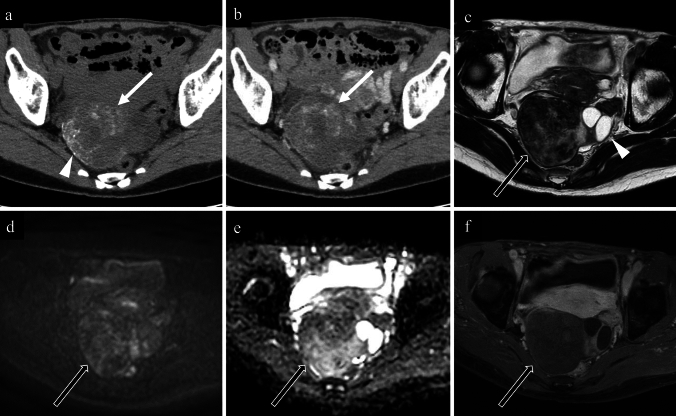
65-year-old woman with a fibroma of the right ovary. a non-contrast CT; b contrast-enhanced CT; c axial T2-weighted image; d axial diffusion-weighted image; e axial apparent diffusion coefficient map; f axial contrast-enhanced T1-weighted image. A round mass is observed on the dorsal side of the uterus, accompanied by amorphous (a; arrow) and punctate calcifications (a; arrowhead). The enhancement is poor, and the contrast-enhanced CT cannot distinguish between the amorphous calcifications and enhancement effects. On T2-weighted imaging, the mass appears as a markedly low-signal solid tumour (a; black arrow) with cysts (c; arrowhead) on its left margin. It shows weak diffusion restriction (c and d; black arrows) and poor enhancement (f; black arrow), consistent with a fibroma. Pathologically, it was diagnosed as a fibroma. The calcification patterns of fibromas varied, and this was the only case exhibiting amorphous calcifications
